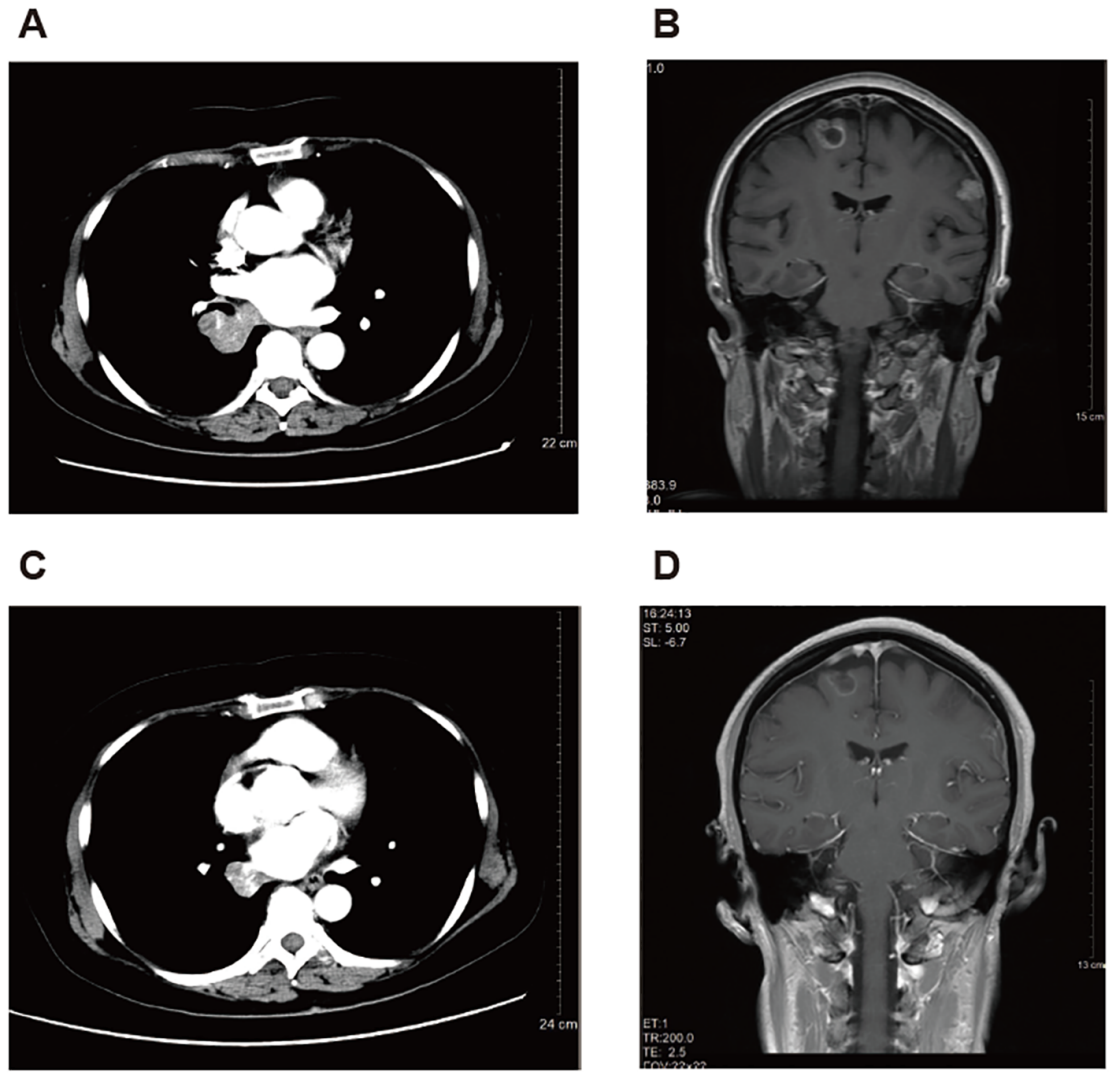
Representative images of computed tomography in a 57-year-old female patient with metastatic non-small cell lung cancer harboring *HER2* exon 17 V659E mutation and amplification treated with later-line trastuzumab deruxtecan. (A, B) Before treatment of trastuzumab deruxtecan. (C, D) After 10-month treatment of trastuzumab deruxtecan.
